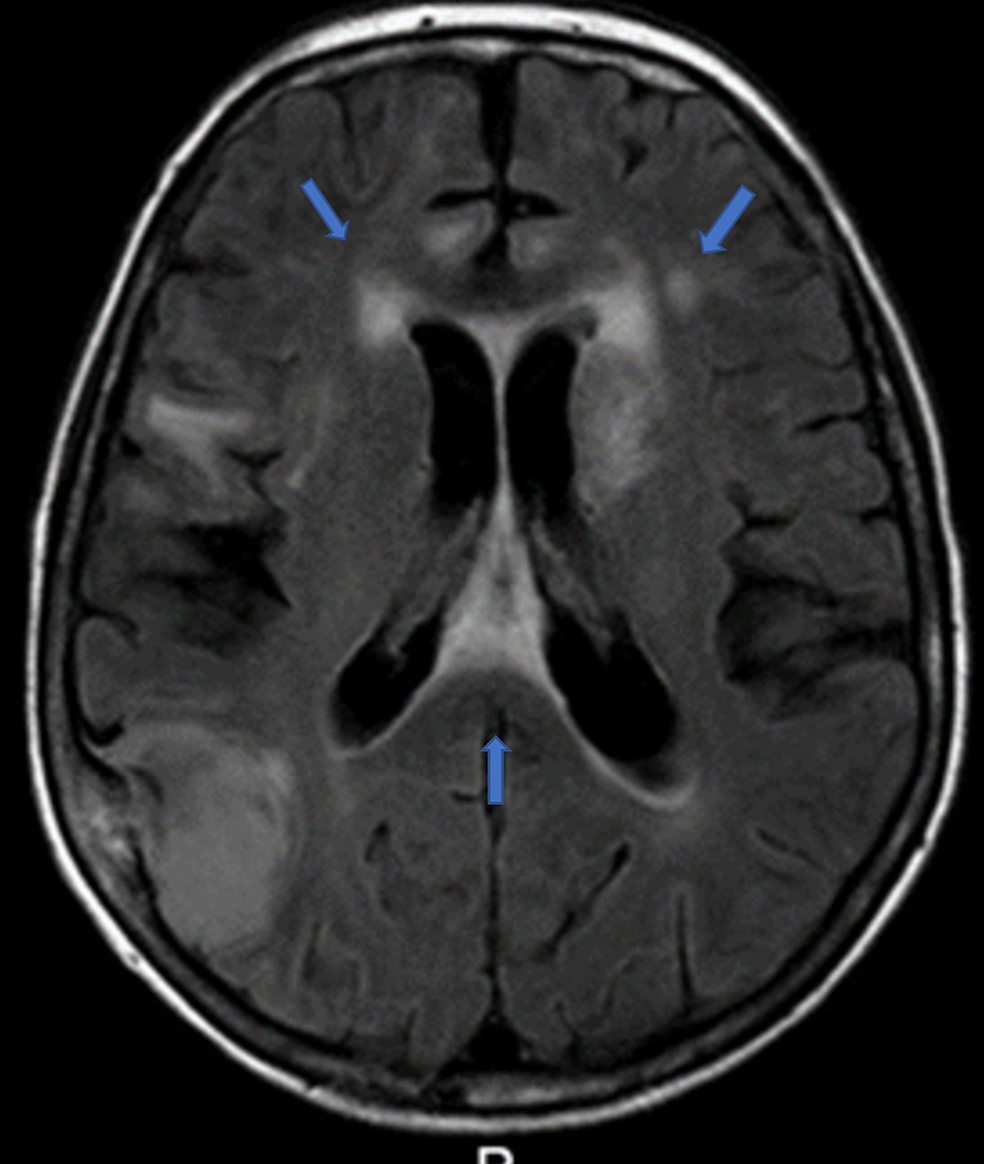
Cranial MRI scan (axial plane) showing hyperintense signal of subcortical white matter - bitemporal regions, mesial temporal lobe, corpus callosum, and internal capsule. Meningioma of the right parietal lobe. MRI - magnetic resonance imaging
